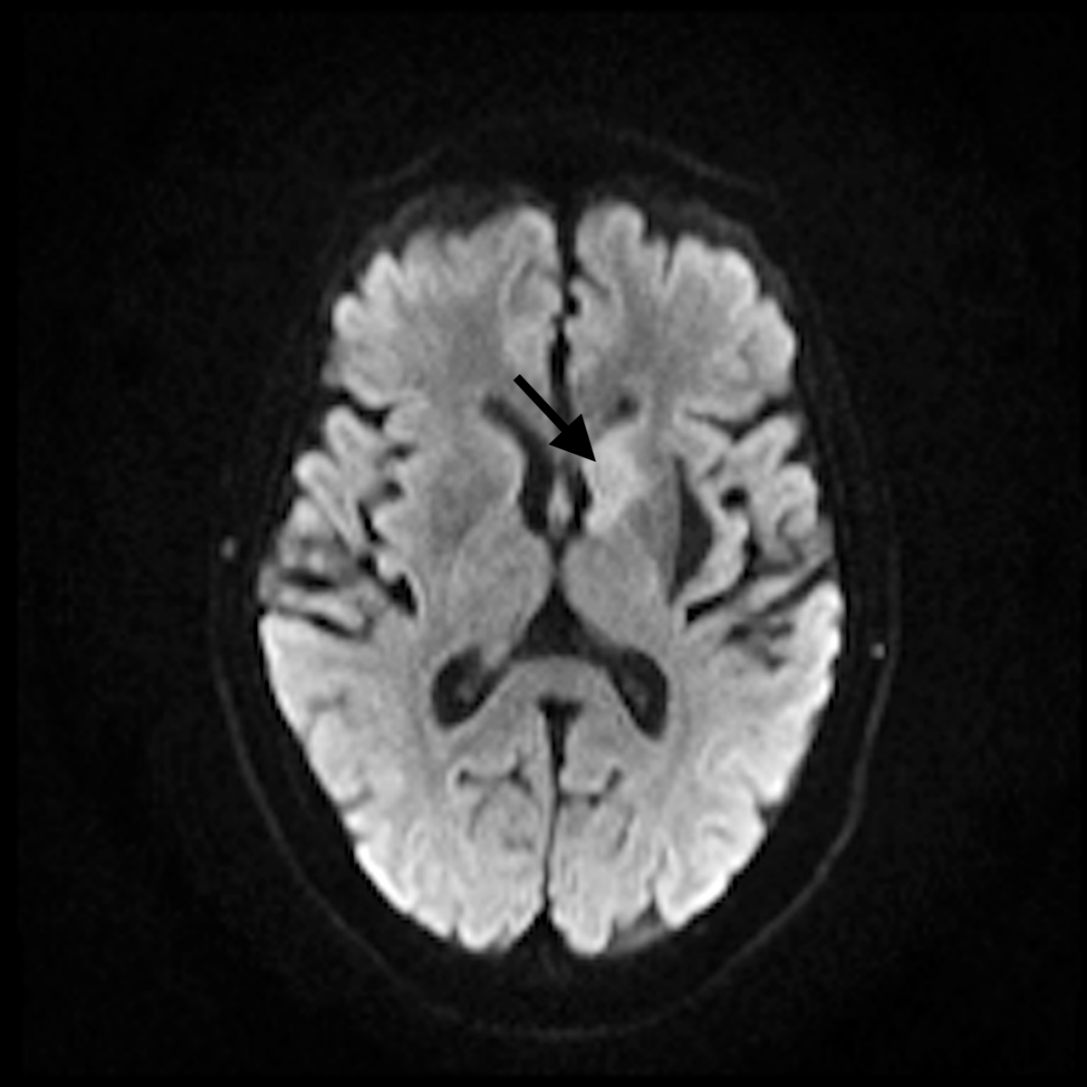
Magnetic resonance imaging (MRI) MRI showing increased signal intensity in the left caudate head on diffusion-weighted imaging (DWI).
